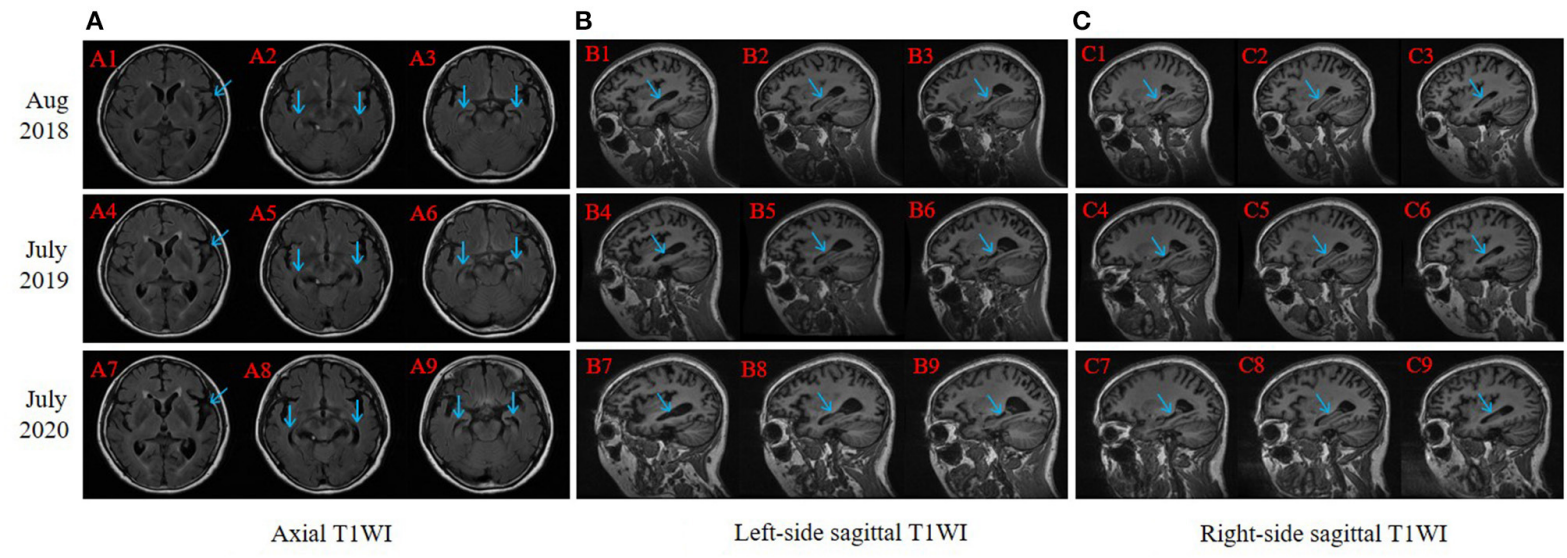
Brain magnetic resonance imaging (MRI) slice-corresponding comparison of the proband for 3 years. Arrows point at progressive atrophy of temporal lobe and hippocampus. A1–A3, B1–B3, and C1–C3 were scanned in August 2018 (2 years after dementia symptom onset in this proband); A4–A6, B4–B6, and C4–C6 were scanned in July 2019; and A7–A9, B7–B9, and C7–C9 were scanned in July 2020. (A) Axial T1WI: each column revealed progressive atrophy of the left-dominant bilateral temporal lobe and hippocampus. (B) Left-side sagittal T1WI: focused on the left part of the hippocampus. (C) Right-side sagittal T1WI: focused on the right part of the hippocampus.
